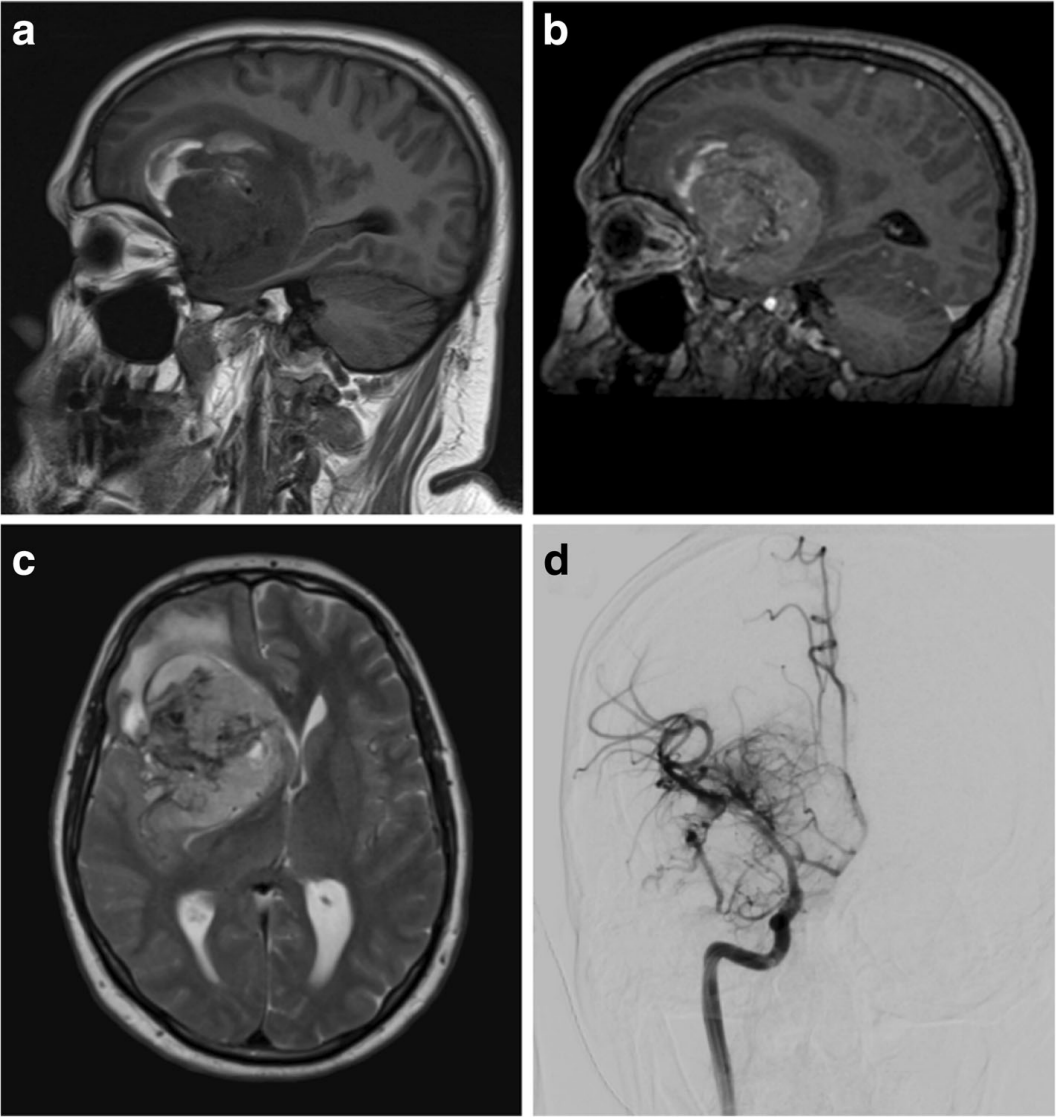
Glioblastoma. a, b Pre- and post-contrast sagittal T1-weighted MR images showing a vascular and heterogeneously enhancing lesion with areas of internal necrosis and haemorrhage. c T2-weighed axial MR image of the same lesion showing internal flow voids, an apparent thin CSF cleft and a small amount of perilesional oedema. d Selective right internal carotid artery angiogram demonstrates a highly vascular mass with multiple abnormal and aneurysmal vessels (performed pre-embolisation)
